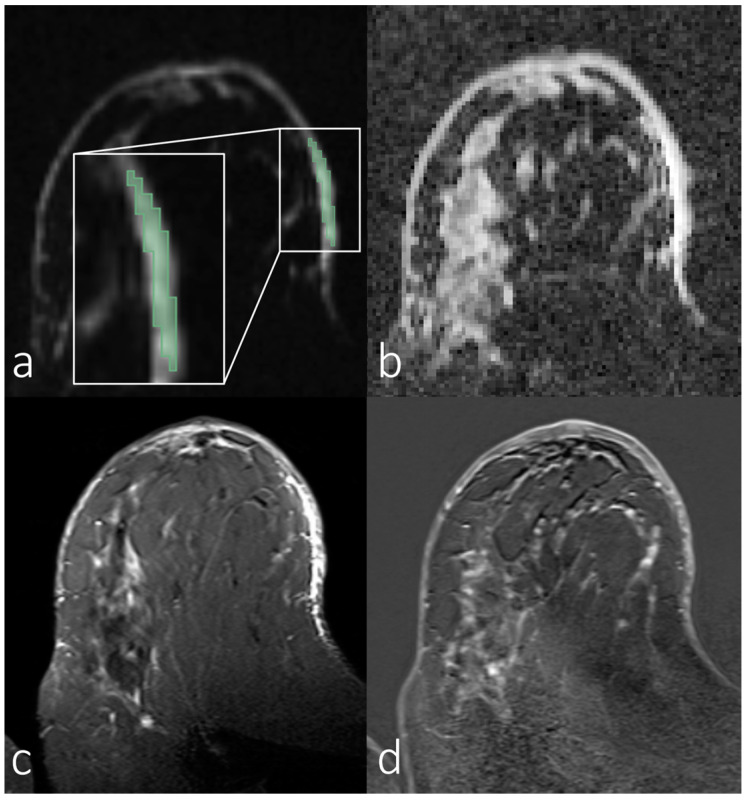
Breast magnetic resonance images of a woman with inflammatory breast carcinoma. (a) Manual segmentation overlaid on the diffusion-weighted image (b = 800 s/mm^2^), with segmentation highlighted in green; (b) apparent diffusion coefficient map; (c) T_2_-weighted image with fat saturation; (d) T_1_-weighted subtraction image after contrast administration.
